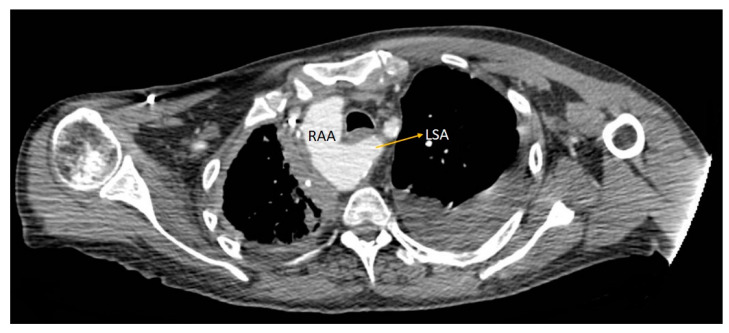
Axial CT image showing type II right aortic arch, with the aberrant origin of the left subclavian artery, emerging from the medial wall of the aortic arch, following a retroesophageal course (orange arrow). RAA—right aortic arch. LSA—left subclavian artery.
